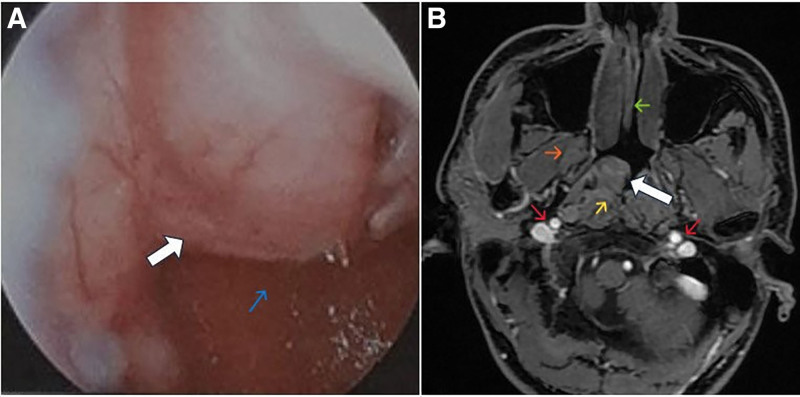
Nasopharyngoscopy (A) and contrast-enhanced axial MRI (B). Large white arrow: nasopharyngeal mass arising from the roof of the nasopharynx with right-sided predominance. (A) Blue arrow: posterior nasopharyngeal wall. (B) Green arrow: nasal septum; red arrows: internal carotid artery and internal jugular vein; orange arrow: medial pterygoid muscle; yellow arrow: nasopharyngeal roof.
